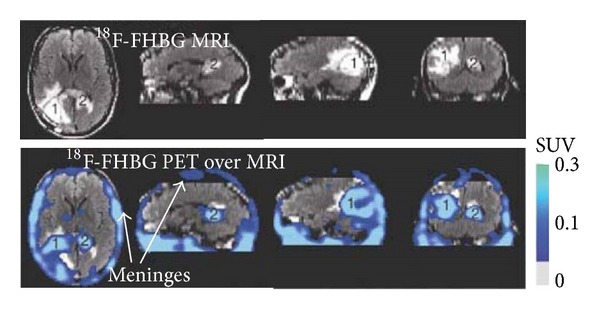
MRI and PET over MRI superimposed brain images of the patient who had been infused with autologous cytolytic T cells expressing IL-13 zetakine and HSV1-tk genes. Images were acquired approximately two hours after ^18^F-FHBG injection. The patient had a surgically resected tumor (1) in the left corner and a new nonresected tumor in the center (2), near corpus callosum of his brain. The infused cells had localized at the site of tumor 1 and also trafficked to tumor 2. ^18^F-FHBG activity is higher than the brain background at both sites. Background ^18^F-FHBG activity is low within the central nervous system due to its inability to cross the blood brain barrier. Background activity is relatively higher in all other tissues. Activity can also be observed in the meninges. The tumor 1/meninges and tumor 2/meninges ^18^F-FHBG activity ratio in this patient were 1.75 and 1.57, respectively. Whereas the average resected tumor site/meninges and intact tumor site to meninges ^18^F-FHBG activity ratio in control patients were 0.86 and 0.44, respectively.
